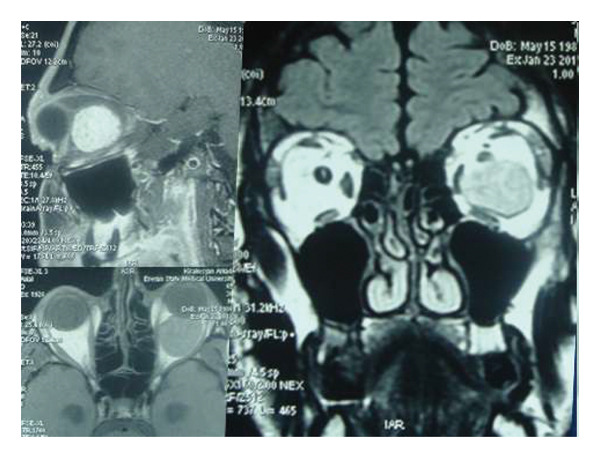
Preoperative MRI of the left orbit. Coronal, axial, and sagittal sections demonstrate a lesion in the left lateral orbit.
